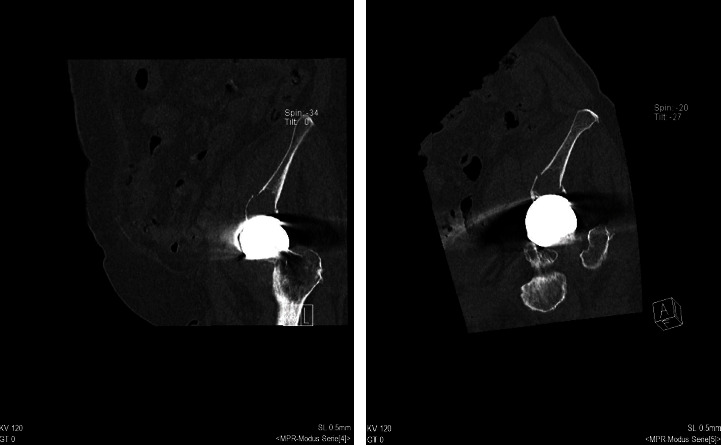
Preoperative CT of the pelvis. (a) shows the large osteolysis in the sagittal plane. (b) is the corresponding coronal plane of the patient's left hip.
